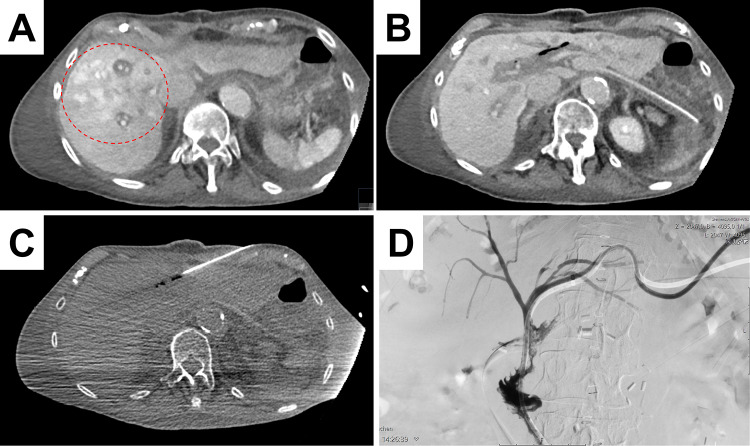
demonstrates the successful placement of a percutaneous transhepatic biliary drainage (PTBD) in the patient from Fig. 2 using the angio CT in hybrid mode. In panel A and B the planning CT for the intervention is shown; the dashed circle(A) highlights an area of focal hyperaemia caused by the numerous puncture attempts performed the previous day. Panel B depicts an optimal left bile duct exhibiting aerobilia and presenting a favorable angle for puncture from the patient’s left side. Panel C shows a CT fluoroscopy image where the needle is being advanced into the bile duct containing aerobilia. Following this, a guidewire was inserted, and a modality switch was performed to conventional angiography. Panel D presents a conventional digital subtraction angiography (DSA) image for confirmation, showing leakage at the anastomosis with drainage into the easy flow drainage system and the correct positioning of the PTBD
